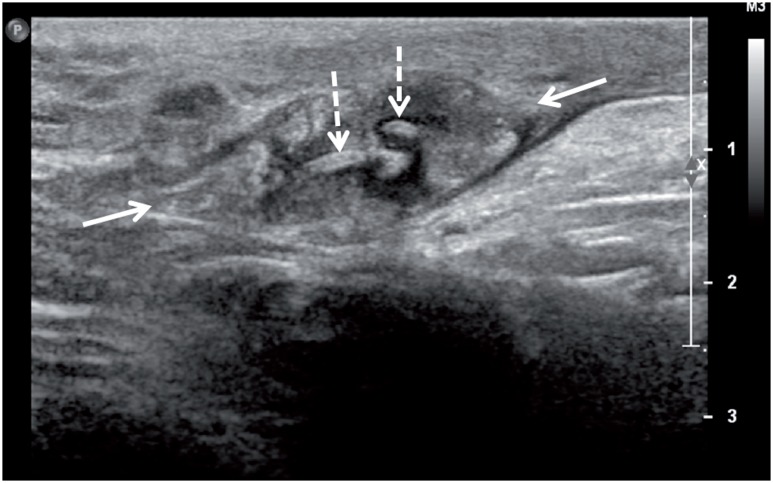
Gray-scale sonography of suture granuloma (arrows). It is observed centrally located curvilinear double echogenicity corresponding to suture material (dotted arrows) to be covered by highly sound attenuating tissue.
